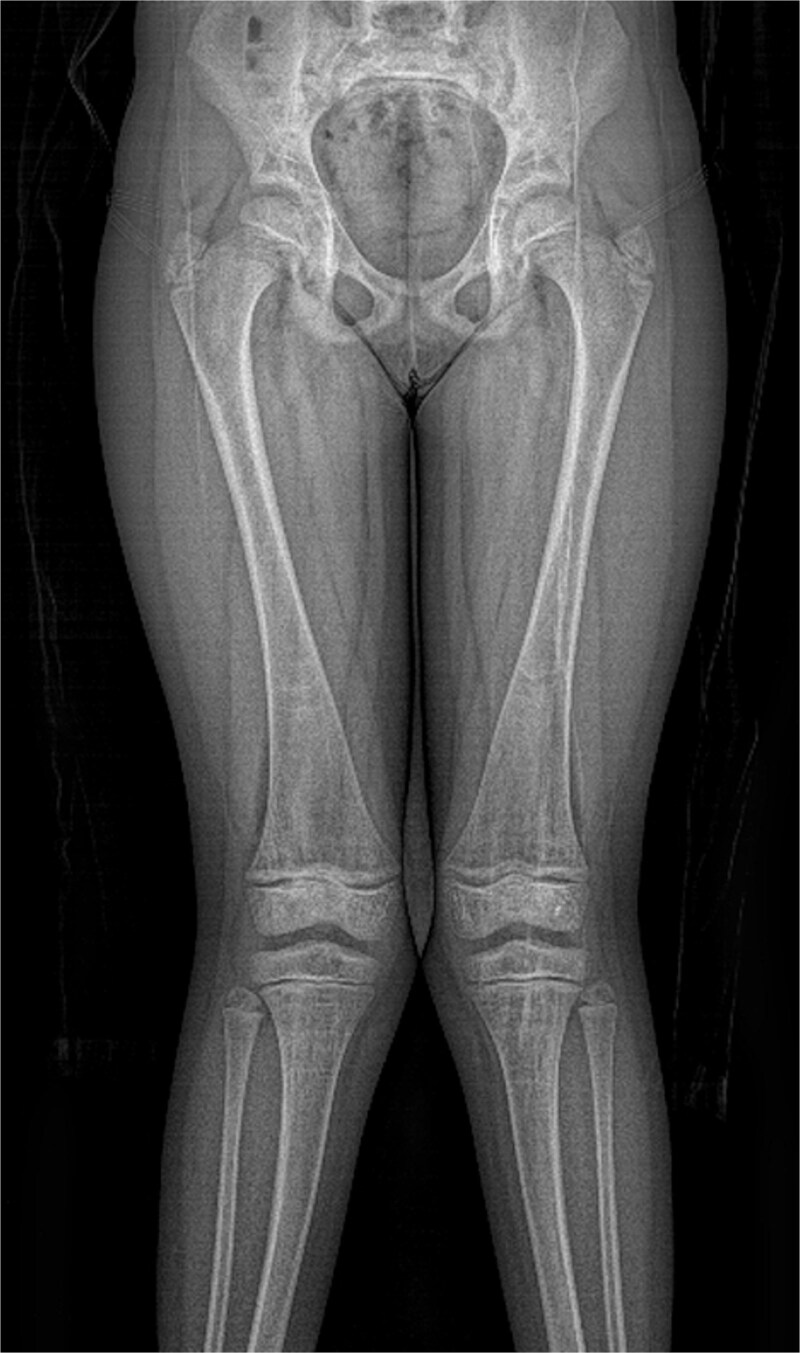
Radiograph demonstrating bowing of both femora and the tibiae. Bilateral coxa Valga.
